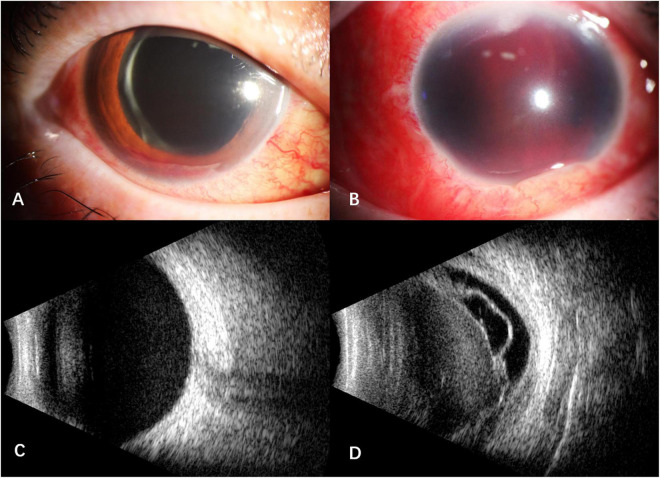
Anterior segment and B -scan ultrasonography of the patient after the fourth intravitreal MTX combined with paracentesis. (A) floating blood cells and a small amount of hyphema in the anterior chamber of the right eye; (B) the anterior chamber was full of blood in the left eye; (C) suspected vitreous hemorrhage in the right eye; (D) suspected vitreous hemorrhage with retinal detachment and proliferative membrane in the left eye.
